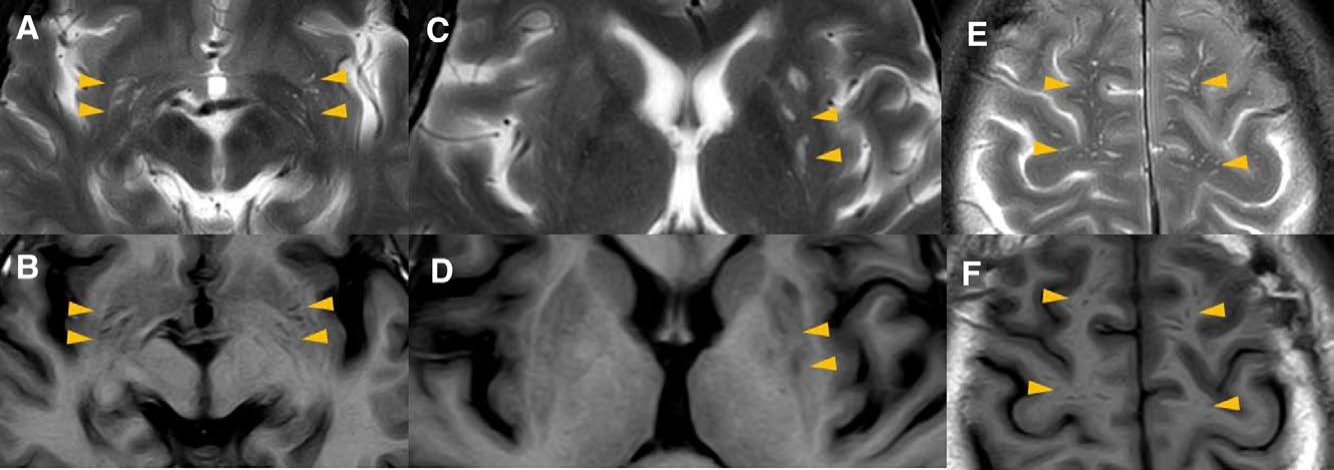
The MRI map during hospitalization. (A–F) Enlarged perivascular spaces in bilateral basal ganglia and perivascular spaces in bilateral centrum semiovale on T2WI and T1WI (yellow arrow heads). MRI = magnetic resonance imaging, T1WI = T1-weight, T2WI = T2-weight.
